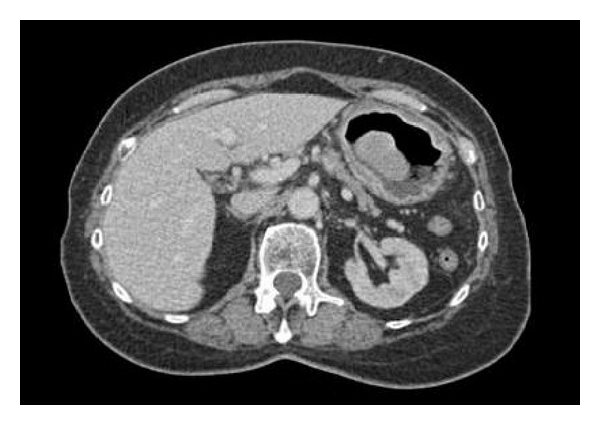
Computed tomography scan. Gastric mass arising from medial wall of stomach.
